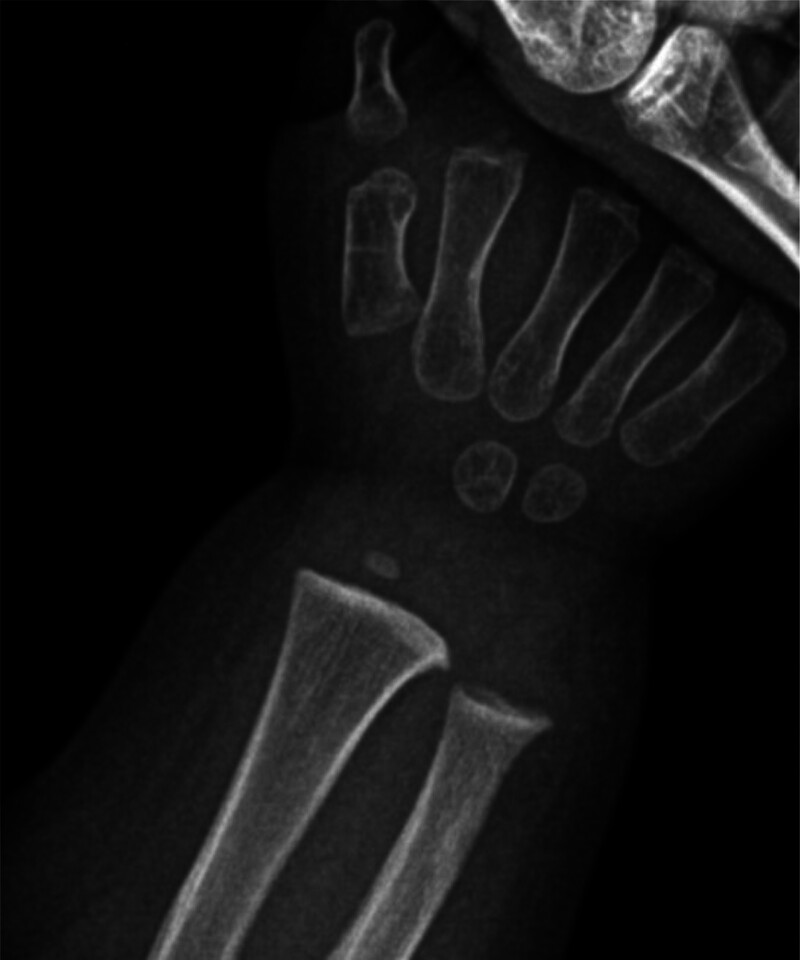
Left wrist X-ray: shortened metacarpals, metaphyseal flaring of distal radius/ulna, osseous remodeling. The left wrist joint and bone changes of the ulna and radius should be evaluated to exclude cartilage dysplasia.
